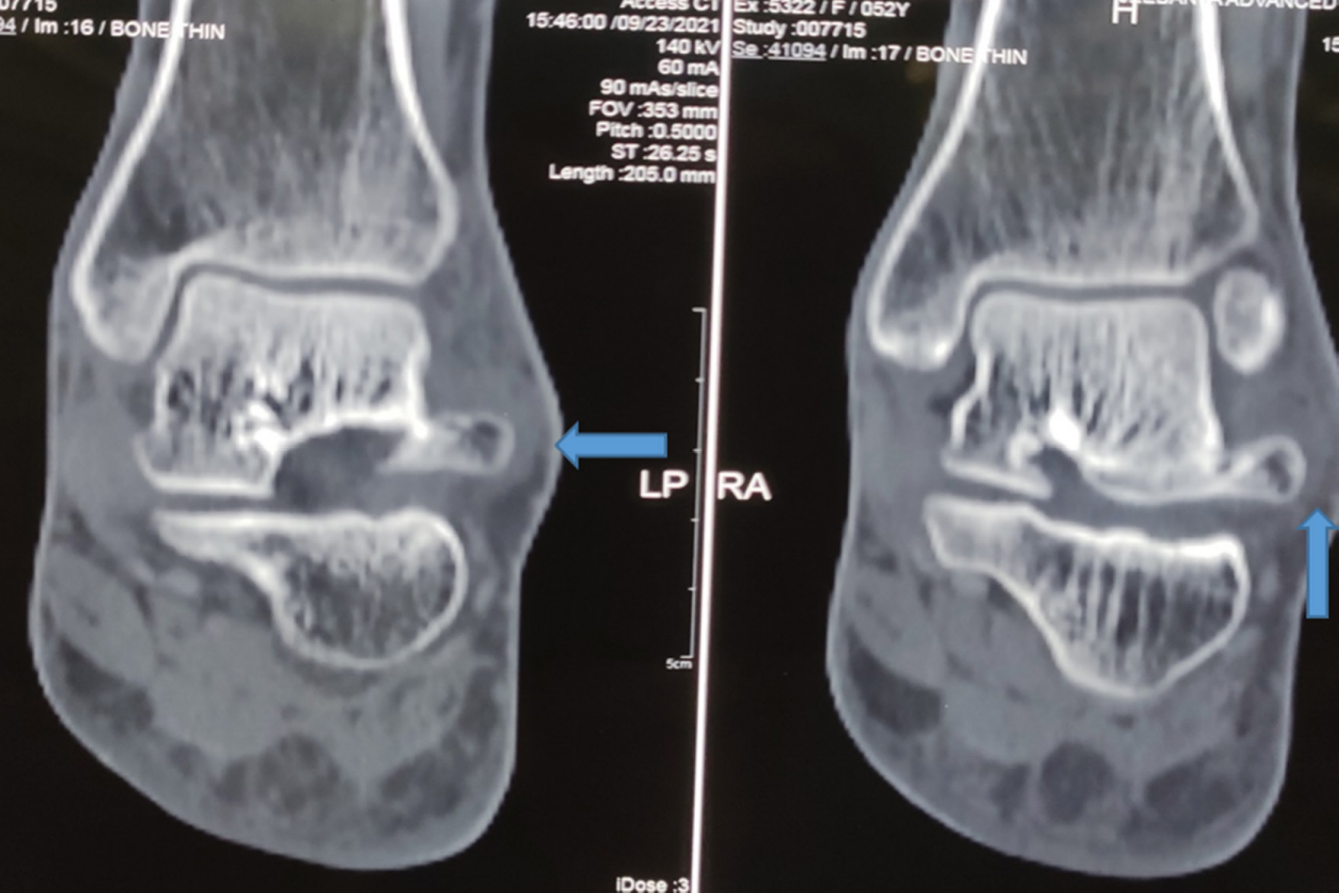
Coronal CT image of left ankle showing a bony outgrowth from the lateral aspect of body talus (arrow)
